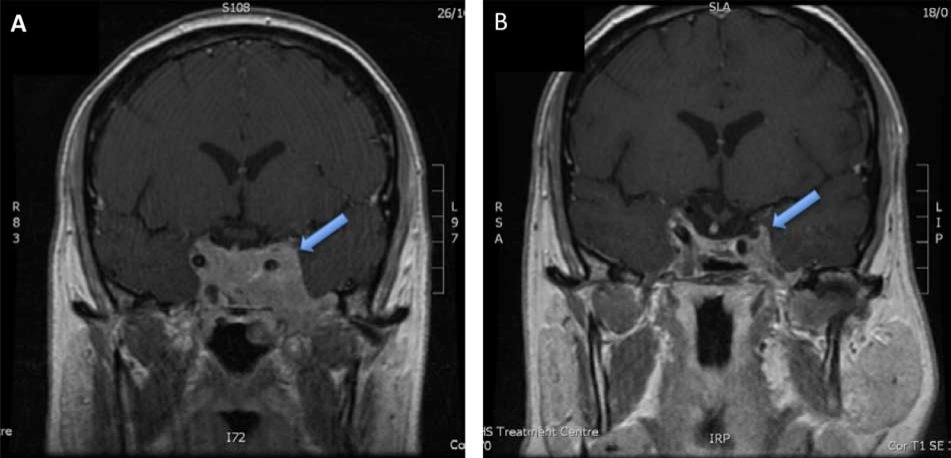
Cranial magnetic resonance imaging scan revealing a large soft tissue mass at the base of the skull (blue arrow, plate A). Following treatment with cabergoline, the soft tissue mass reduced in size significantly after 3 months of therapy (blue arrow, plate B).
